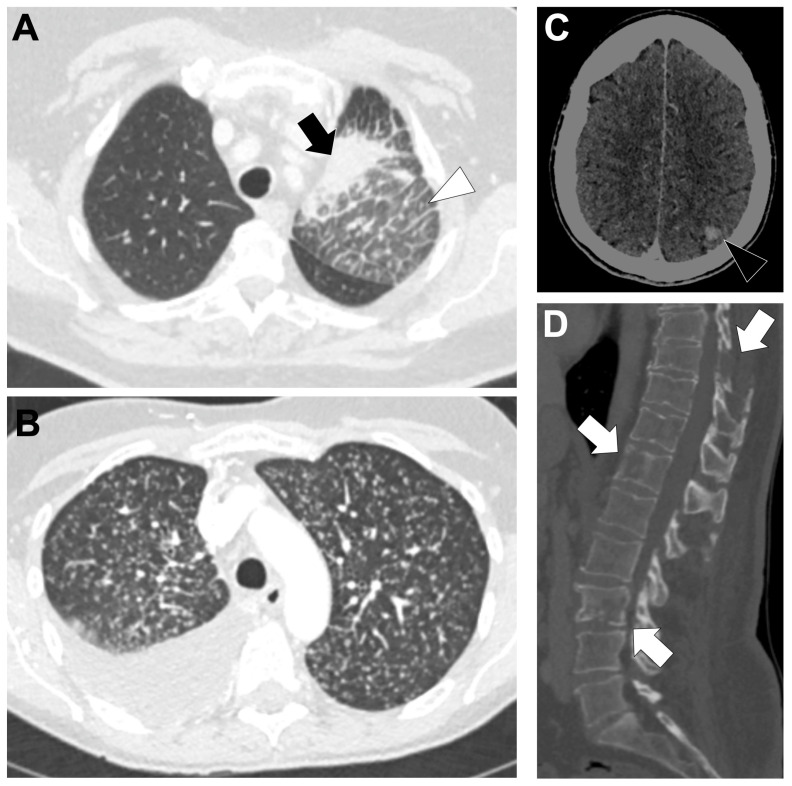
Representative baseline CT imaging findings in patients with EGFR-altered lung adenocarcinoma (LUAD). (A) A 71-year-old never-smoking woman presenting with a spiculated mass in the left upper lobe (black arrow), surrounded by ground-glass opacities and associated lymphangitic carcinomatosis (white arrowhead). Molecular analysis identified an exon 21 L858R EGFR mutation. (B–D) A 51-year-old woman presenting with a diffuse miliary pulmonary pattern and pleural effusion (B), a brain metastasis located in the left parietal cortex (black arrowhead) (C), and multiple lytic vertebral bone metastases (white arrows) (D). Molecular analysis revealed an exon 19 deletion.
